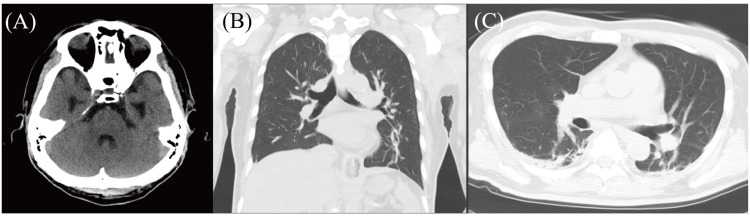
Postmortem computed tomography (CT) imaging (A) No brainstem lesions or fresh cerebral infarctions were detected in any areas of the brain. (B, C) No food or other foreign body was noted in the main bronchus or trachea.
